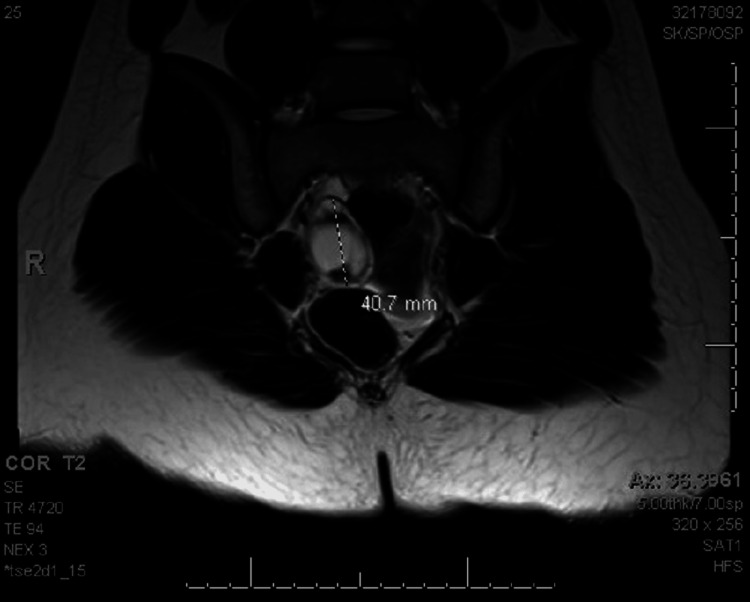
MRI of the abdomen with and without contrast demonstrating one of the masses discovered on initial presentation in 2016.
